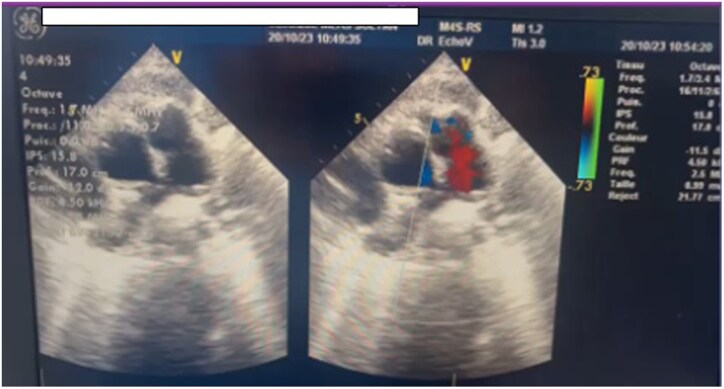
Postoperative transthoracic echocardiography showing no residual pulmonary artery aneurysm.
